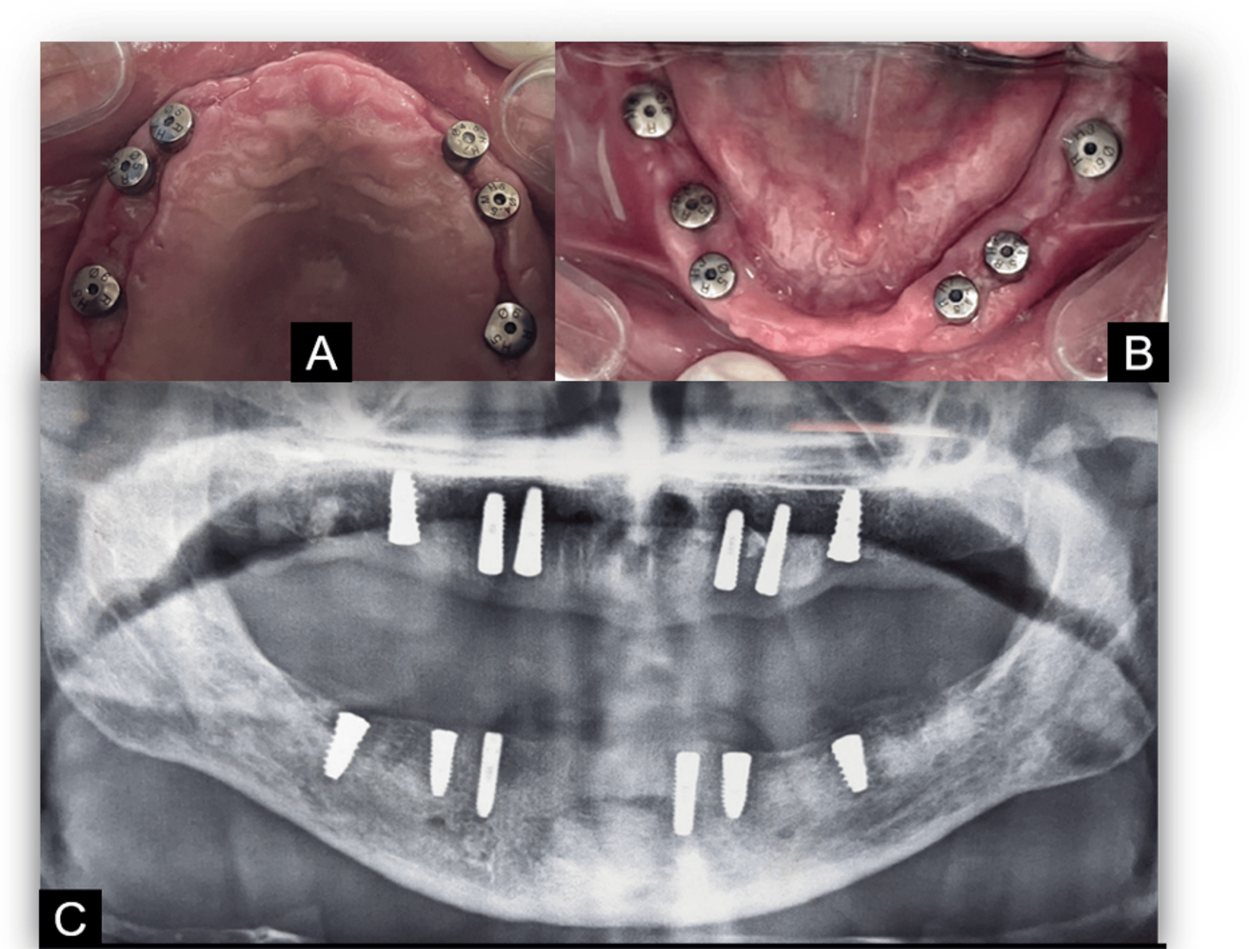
Postoperative images (A) Maxillary arch (six implants); (B) mandibular arch (six implants); (C) postoperative radiograph
